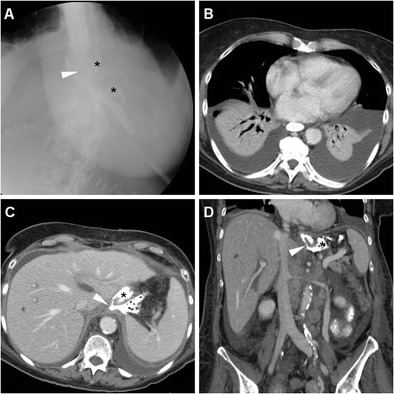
In a 69-year-old woman with advanced carcinoma of the gastric body, palliative total gastrectomy was performed. Five days after surgery, CF (A) showed patent EJS with opacification of extensive anastomotic leakage (*). A few days later, on conservative management, contrast-enhanced CT (B-D) showed bilateral pleural effusions and atelectasis (B), patent EJS (arrowheads) and persistent leak (*)
